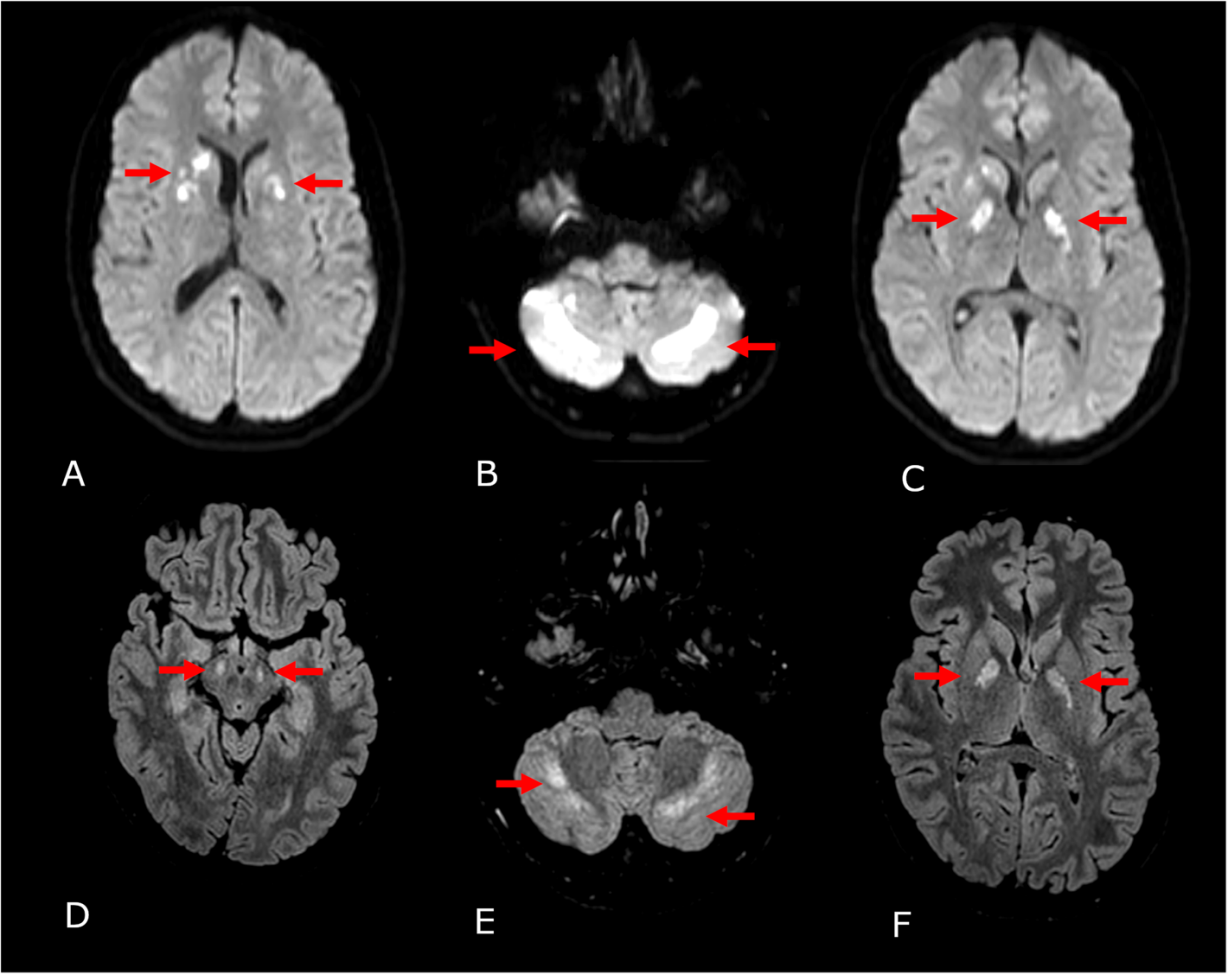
MRI imaging on day 1 and day 11 after intoxication. Timepoint 1 (Day 1 after intoxication) DWI sequences: a 1 basal ganglia lesions; b Bilateral cerebellar lesions; c Capsula interna lesions, posterior limb. Timepoint 2 (Day 11 after intoxication) FLAIR sequences: d new lesion at timepoint 2 in crura cerebri; e cerebellar lesions; f Capsula interna lesions, posterior limb
